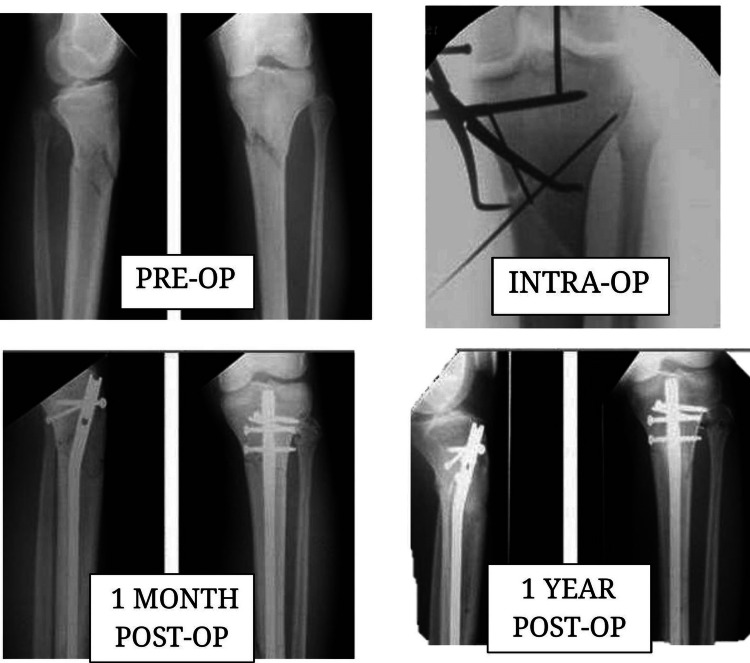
Representative IMILN case with Poller-screw adjunct Top left: preoperative anteroposterior and lateral radiographs (PRE-OP). Top right: Intraoperative fluoroscopy demonstrating Poller (blocking) screw placement to neutralize apex-anterior tendency during nail insertion; a proximal-lateral entry point was used. Bottom left: one-month postoperative radiographs after interlocking nail fixation with Poller-screw augmentation. Bottom right: 12-month follow-up radiographs demonstrating complete radiological union with maintained alignment IMILN: intramedullary interlocking nailing
